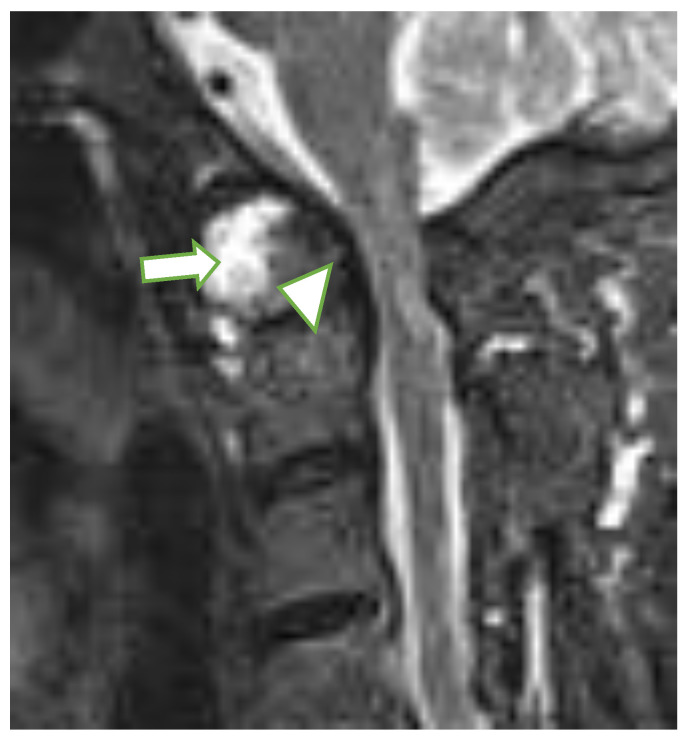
Sagittal MRI, T2-w TIRM image in a 65-year-old female with rheumatoid arthritis shows dens erosions (arrowhead) and periodontal effusion and pannus (long arrow). MRI: magnetic resonance imaging, TIRM: turbo inversion recovery magnitude.
